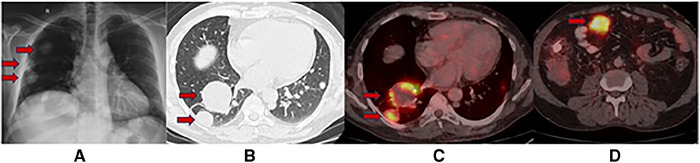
(A) Chest radiograph posteroanterior view shows multiple lung metastases (arrows). (B) Computed tomography (CT) axial image of the thorax shows multiple lung metastases (arrows). (C) 18F-fluorodeoxyglucose (FDG) positron emission tomography (PET)/CT axial image of the thorax shows multiple FDG-avid lung metastases (arrows). (D) 18F-FDG PET/CT axial image of the abdomen shows FDG-avid peritoneal metastasis (arrow).
